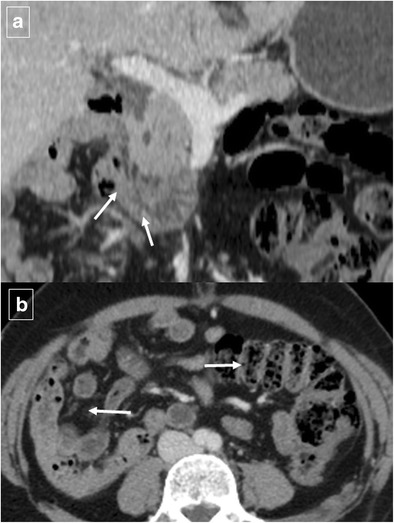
Gastrointestinal nonrotation in a 46-year-old woman. a Reformatted coronal contrast-enhanced CT image shows that the third portion of the duodenum (arrows) does not cross the midline of the abdomen. b The small bowel is located in the right side of the abdomen and the colon in the left side (arrows) on axial CT image
